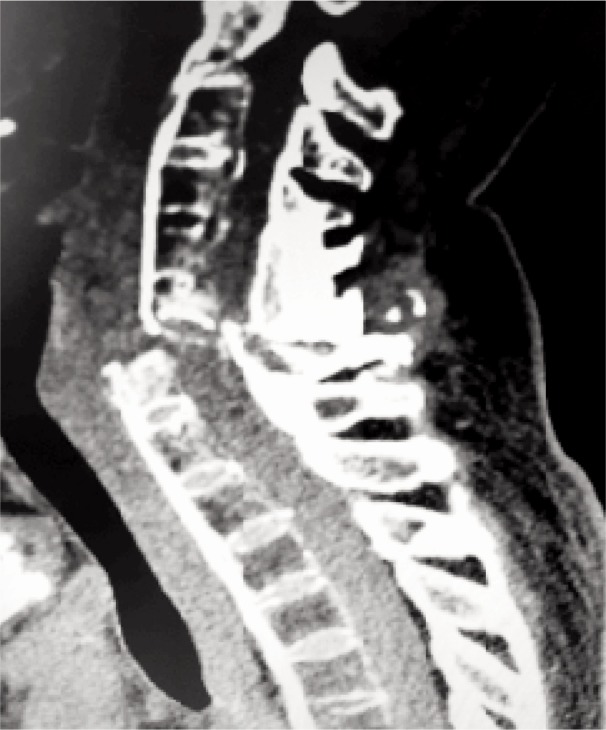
CT cervical spine: sagittal view showing fracture-dislocation of C6 vertebra with facet joint subluxation at C6/C7.
